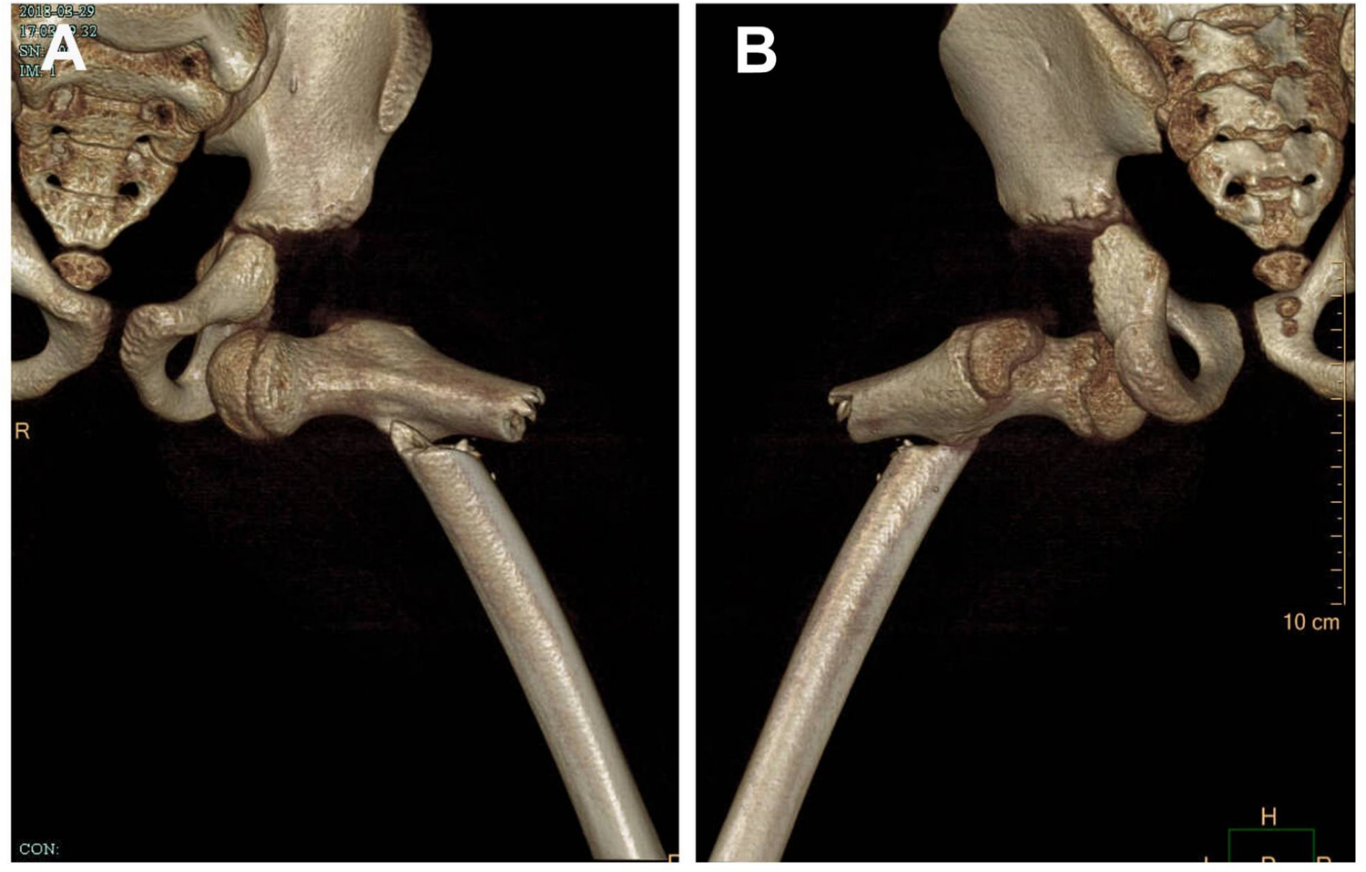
Three-dimensional CT scan of the hip of an 8-year-old child. (A) Anterior observation. (B) Posterior observation.
